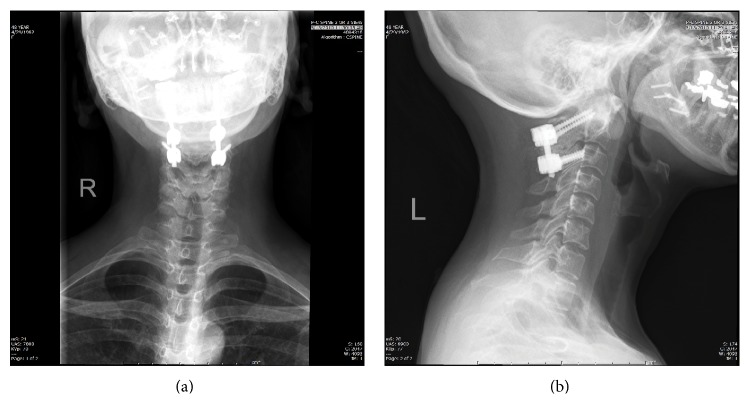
AP (a) and lateral (b) X-rays depicting a C1-2 fusion using C1 lateral mass and C2 pars screws.
